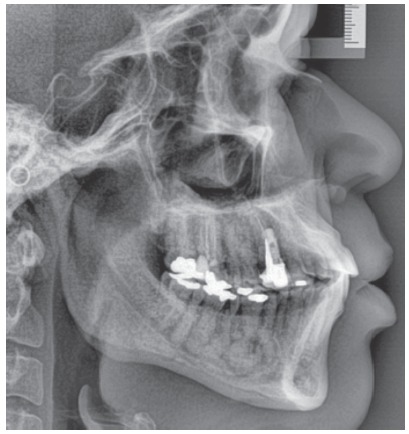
Other radiographic findings of same clinical case with florid cemento-osseous dysplasia seen for evaluation of possible orthodontic treatment. Mandibular lesions are mixed and overlap roots of molar teeth, as they are randomly distributed in posterior region; cotton-wool appearance.
